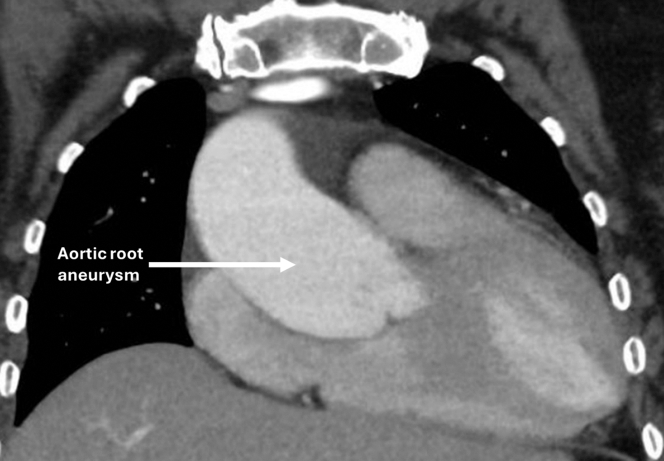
Computed Tomography of the Chest Demonstrating a Dilated Aortic Root of 5.3 cm
